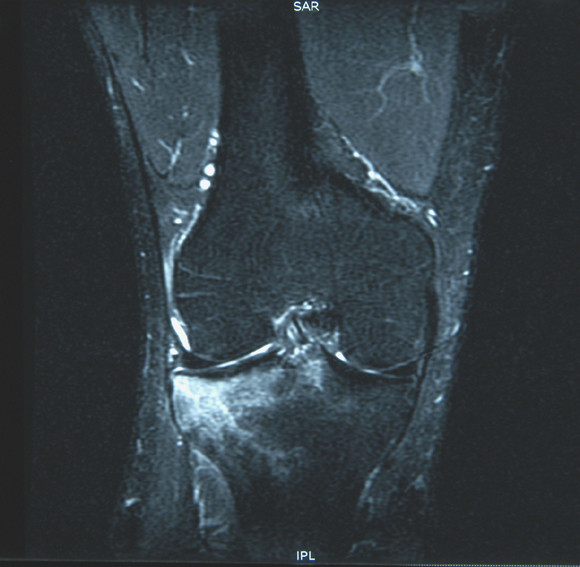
Coronal STIR sequence MRI demonstrating extensive bone marrow oedema within the lateral tibial plateau extending to the articular cartilage.
